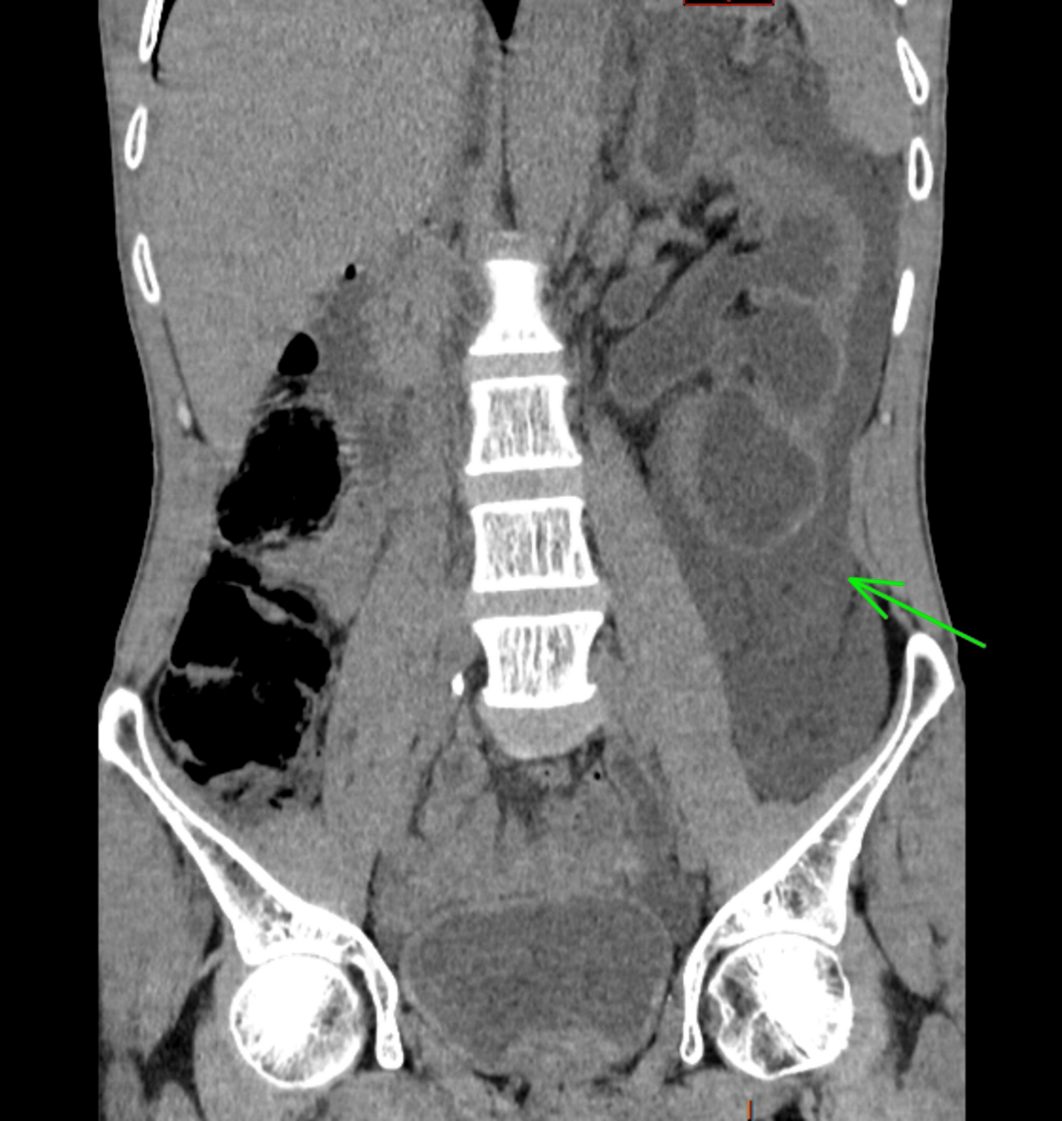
Coronal section of a CT of the abdomen revealing perinephric collection (green arrow).
